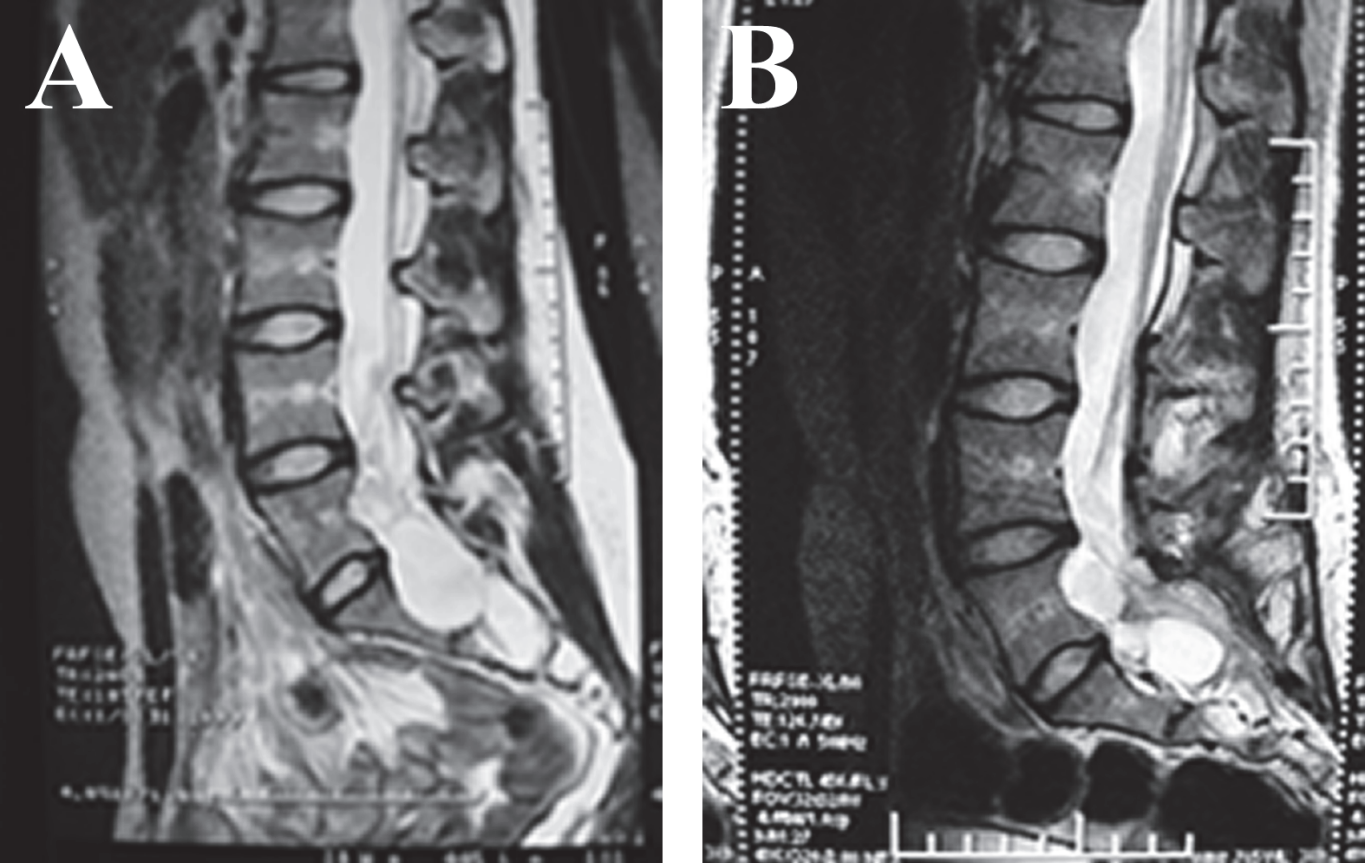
MRI study of shrinkage of the cyst. A. Sagittal T2WI MRI shows multiple cysts before fibrin gel therapy. B. Sagittal T2WI MRI obtained 18 months after operation shows that the cysts became smaller but did not disappear completely.
